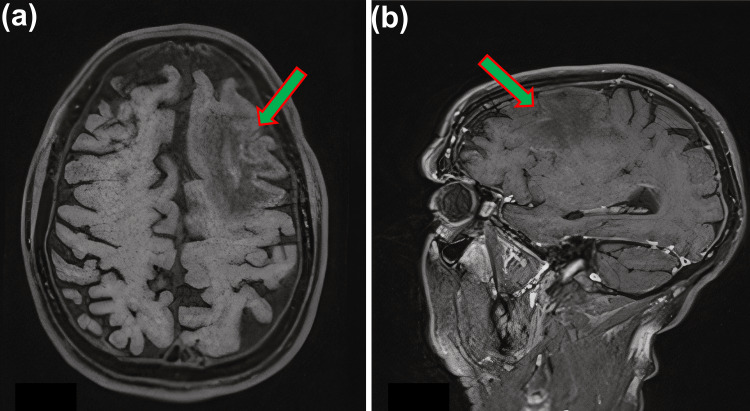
MRI images (a) T1 image without contrast, and (b) T1 image with contrast (sagittal view). These MRI images show a focal signal abnormality predominantly in the left frontal lobe white matter with subjective mild contrast enhancement within a left high frontal sulcus.
